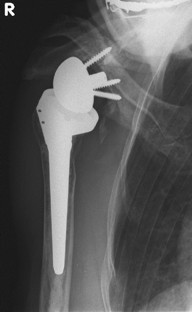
Antero-posterior radiograph of a 64 year-old female patient ´ s right shoulder with an implanted inverted total shoulder prosthesis Delta at 39 months of follow-up. Radiological analysis reveals "grade 4 = erosion over the inferior screw with extension under the base plate" of infraglenoidal scapular notching according to Nerot.
